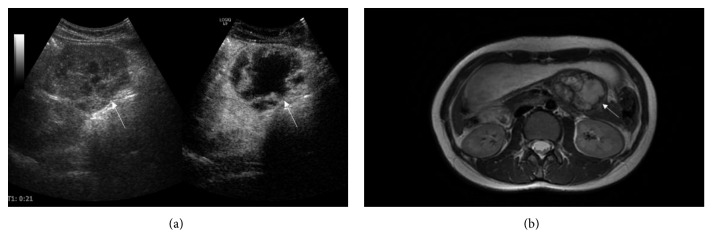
A 17-year-old woman with a SPT in the pancreatic tail. (a) CEUS shows the ring-shaped enhancement of the tumor and the solid components are enhanced separately. (b) Enhanced T2-weighted MR image shows the lesion with capsule, which is progressively enhanced.
